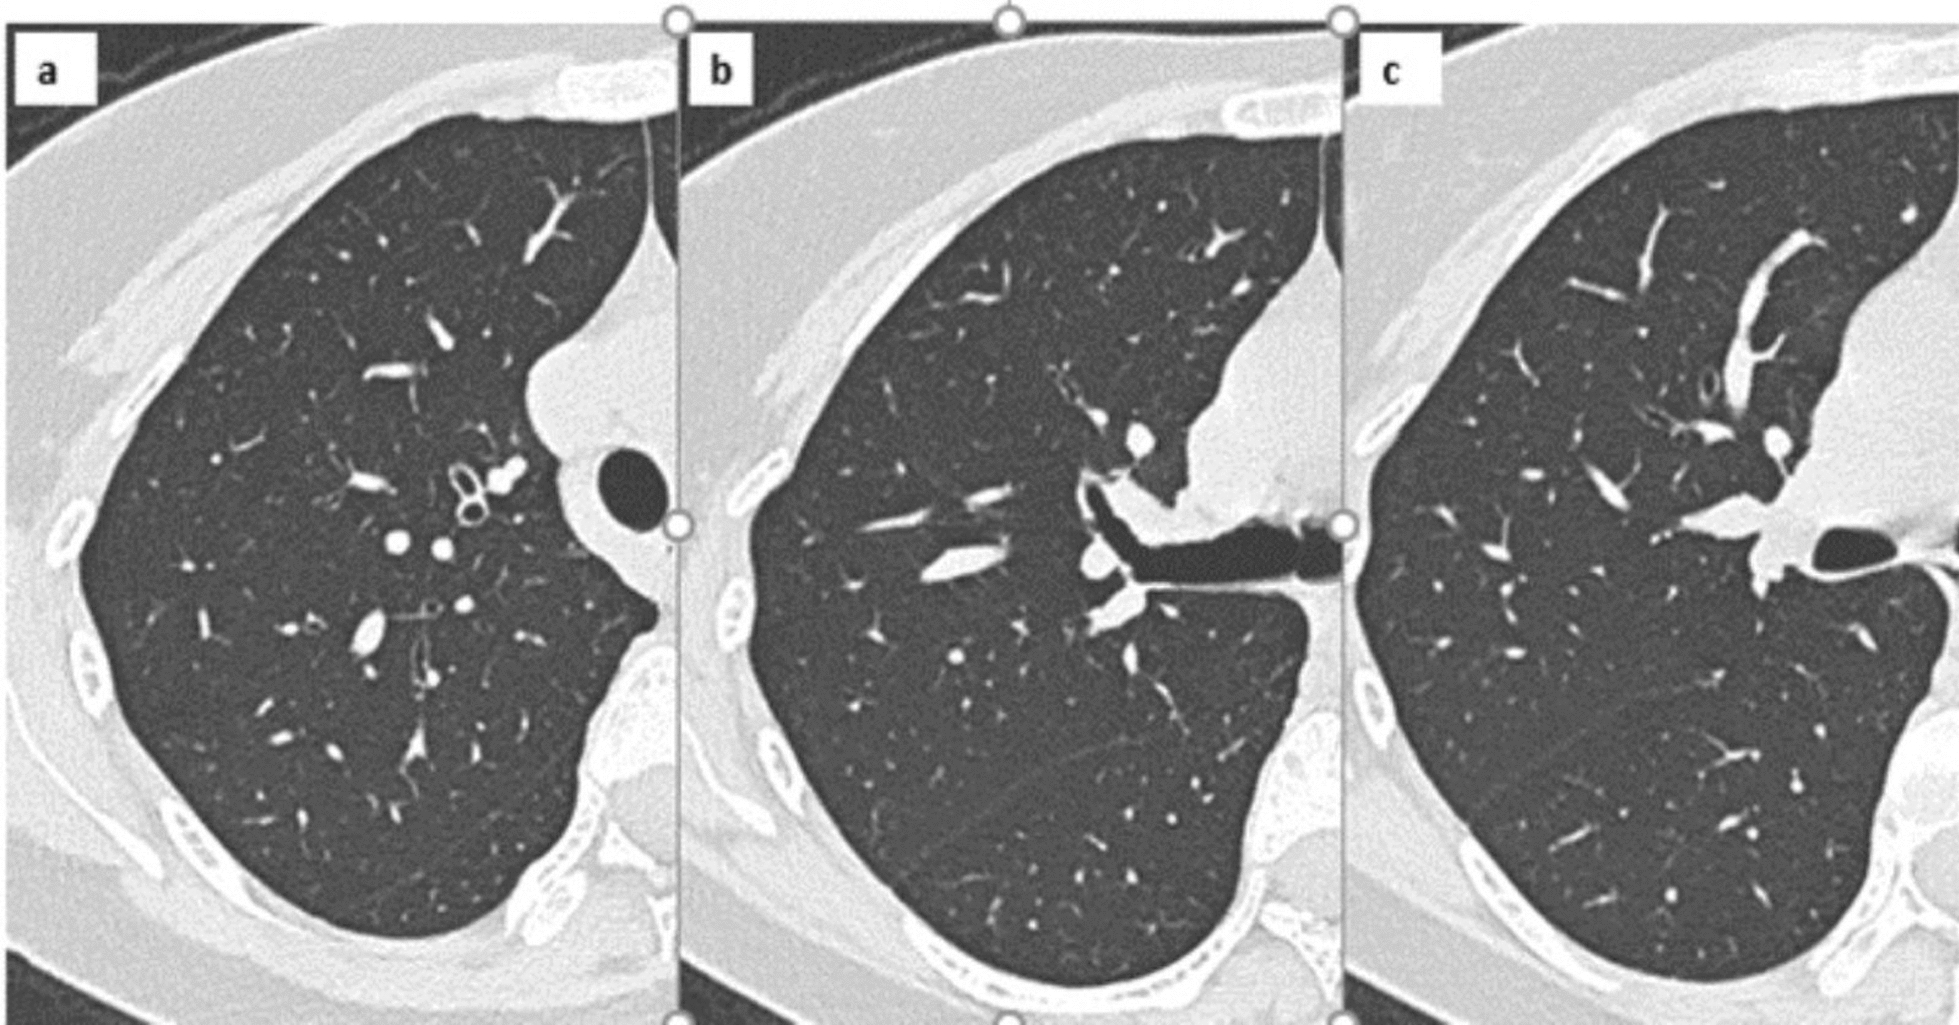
The non-contrast computed tomography scan performed after 7 months shows complete resolution of the pulmonary findings
